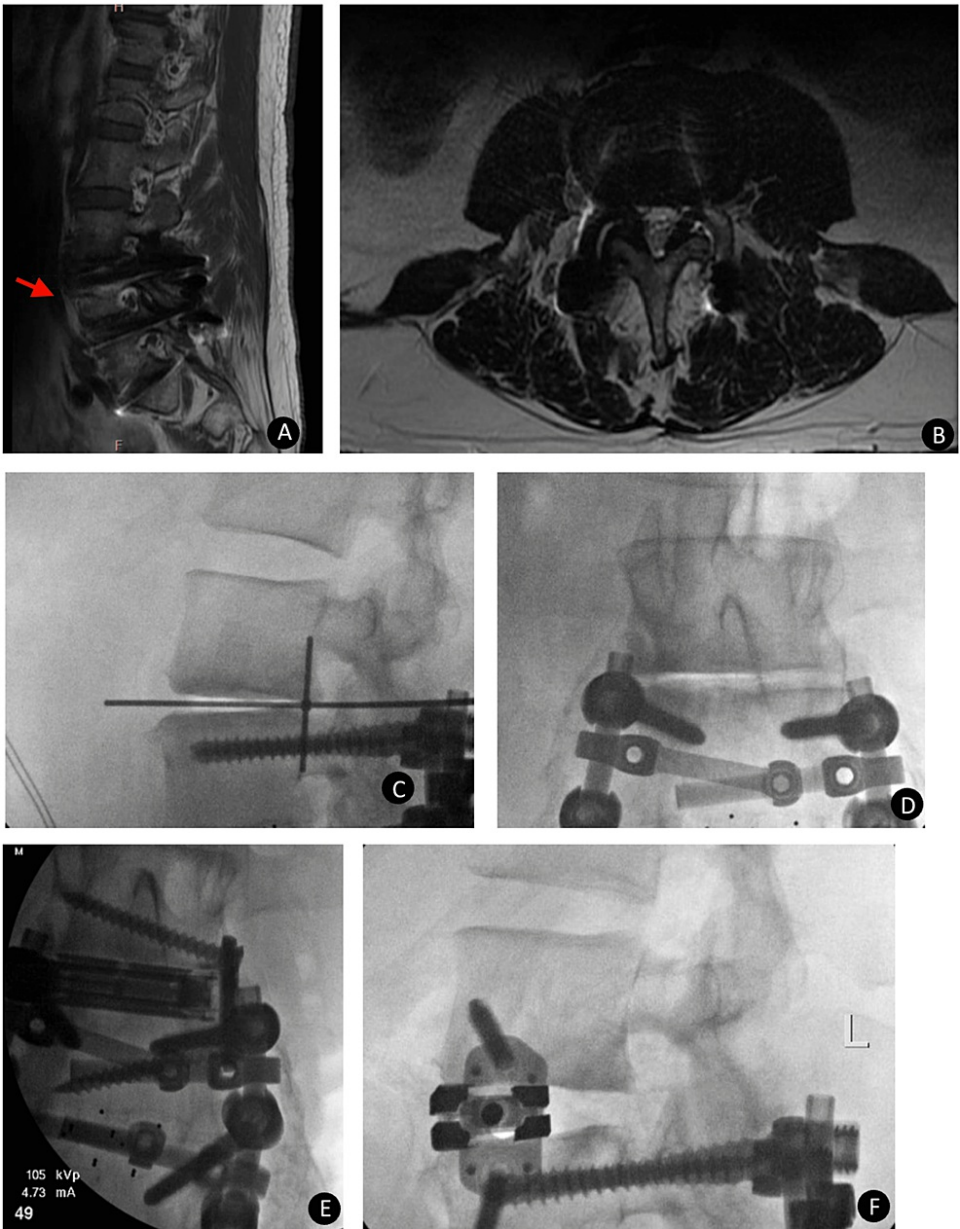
Case 4 postoperative radiographic evaluation of dual expandable spacer and screw placement A 55-year-old male with radiculopathy, neurogenic claudication, and low back pain. Preoperative sagittal (A) and axial (B) MRI showing L3-4 central canal and foraminal stenosis with severe adjacent level disc degeneration (red arrow). Intraoperative lateral (C) and anteroposterior (AP) (D) x-ray images showing steps in the placement of posterior fusion hardware. Intraoperative AP (E) and lateral (F) x-ray images show an expanded interbody spacer with maintained disc space height and no subsidence.
